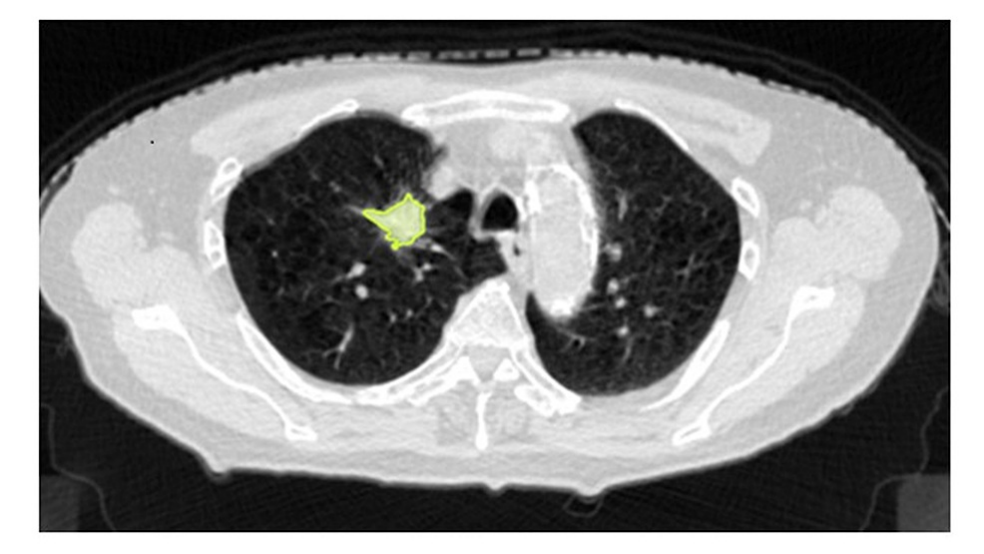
Contour extraction for SBRT An automatic contouring function installed in Velocity software was used for acquiring tumor volume in this study. A threshold value of a CT value was -250 HU. In addition, one experienced radiation oncologist excluded blood vessels and chest walls manually.
